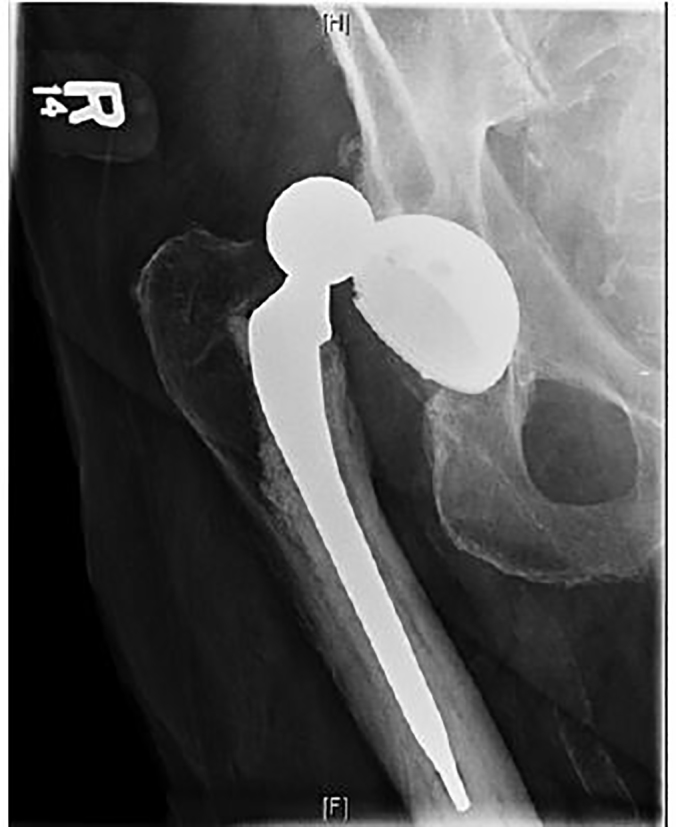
Anterior-posterior radiograph of the patient’s right hip demonstrating the hip prosthesis dislocated superiorly. Lateral films (not shown) indicated posterior displacement as well.
